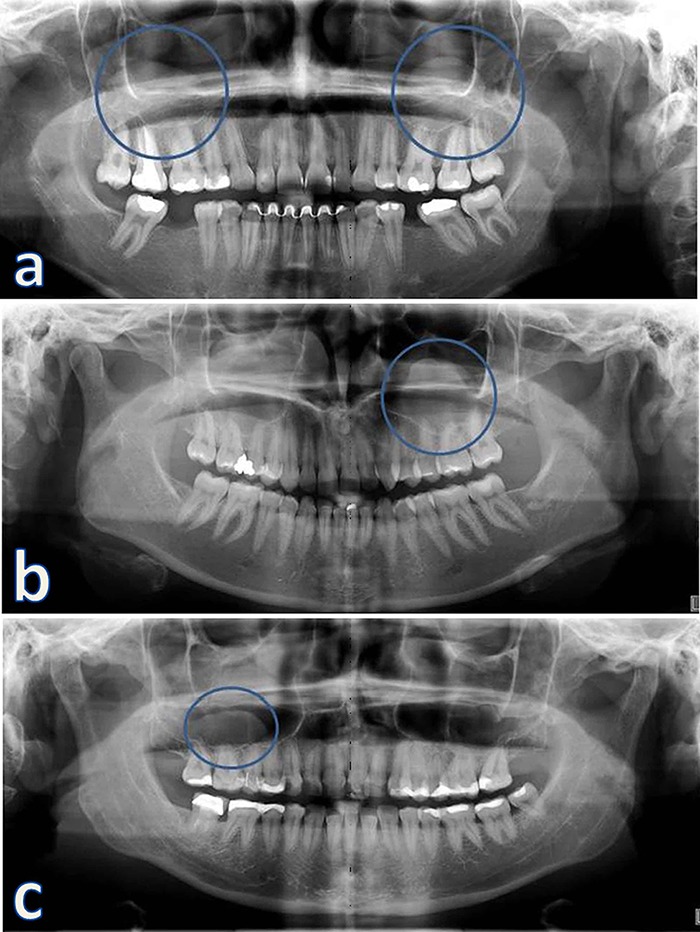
Panoramic radiographs; with images suggestive of a: bilateral mucous retention cyst (circles); b: unilateral mucous retention cyst of the left maxillary sinus and c: unilateral mucous retention cyst of the right maxillary sinus.
